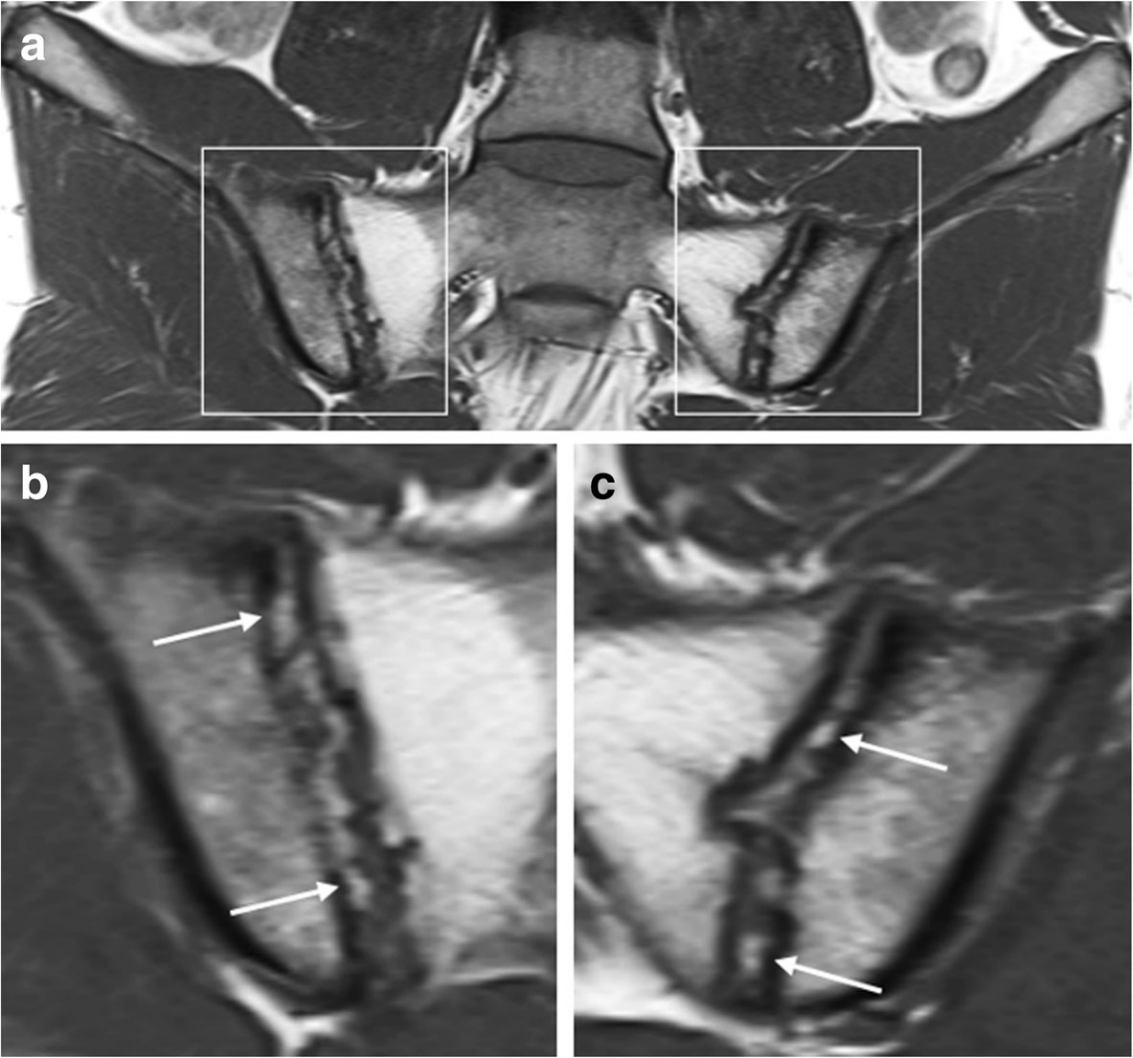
Intra-articular high signal intensity in the sacroiliac joints space on T1-weighted MR images. Coronal oblique T1-weighted MR images acquired in a 25-year-old man with SpA show high intra-articular signal intensity (arrows). This high signal intensity is clearly filling up the eroded iliac bone on the right (i.e., “backfill”). Extensive fatty degeneration of the bone marrow of the sacral side of the sacroiliac joints is present, i.e., post-inflammatory structural changes. Note: b and c are enlargements of the regions outlined in a
